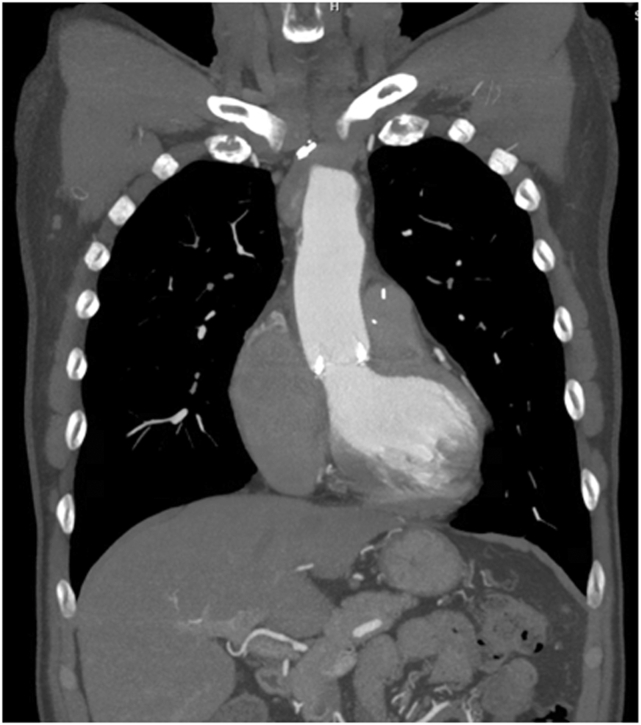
Postoperative CT scan. Coronal image showing SAVR and complete removal of calcium by Excalibur. *CT*, Computed tomography; *SAVR,* surgical aortic valve replacement.
